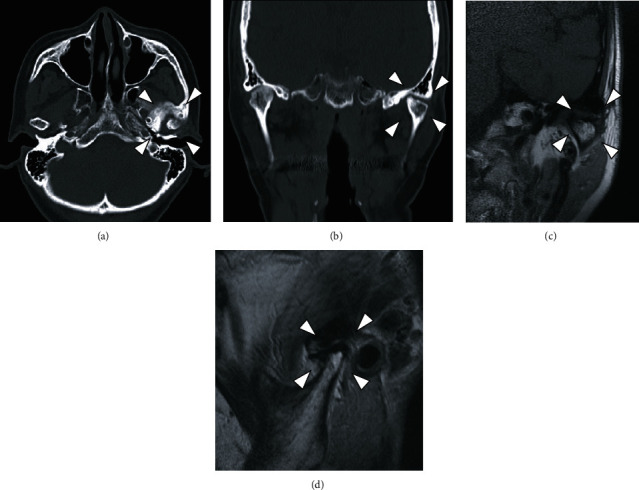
CT and MR images approximately 2 years after the surgery: (a, b) CT images—(a) axial image and (b) coronal image; (c, d) MR images, T1-weighted image. None of the images suggest recurrence.
